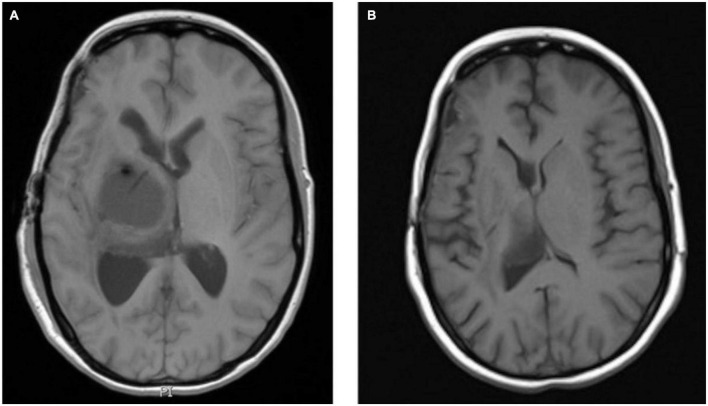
Axial T1 MRI images ganglioglioma pre-BRAF therapy (A) and 3 months post-BRAF therapy (B).
